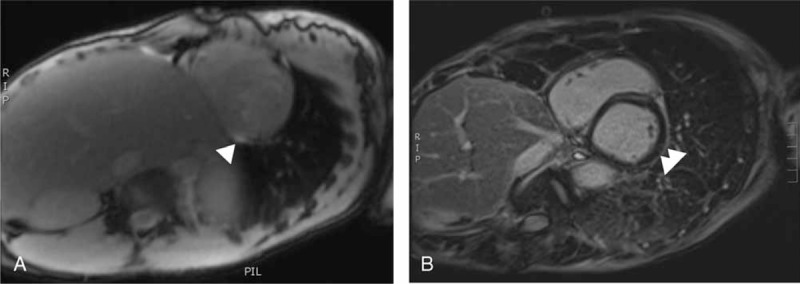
Example of the cardiac-MRI features suggestive of myocarditis. (A) White arrow: myocardium spontaneous T2-hypersignal of the left ventricle consistent an inflammatory process (edema, patient 11). No pericardial effusion. (B) White arrow: myocardium late-T1-post-gadolinium enhancement of the left ventricle consistent an inflammatory process. No pericardial effusion (patient 7).
